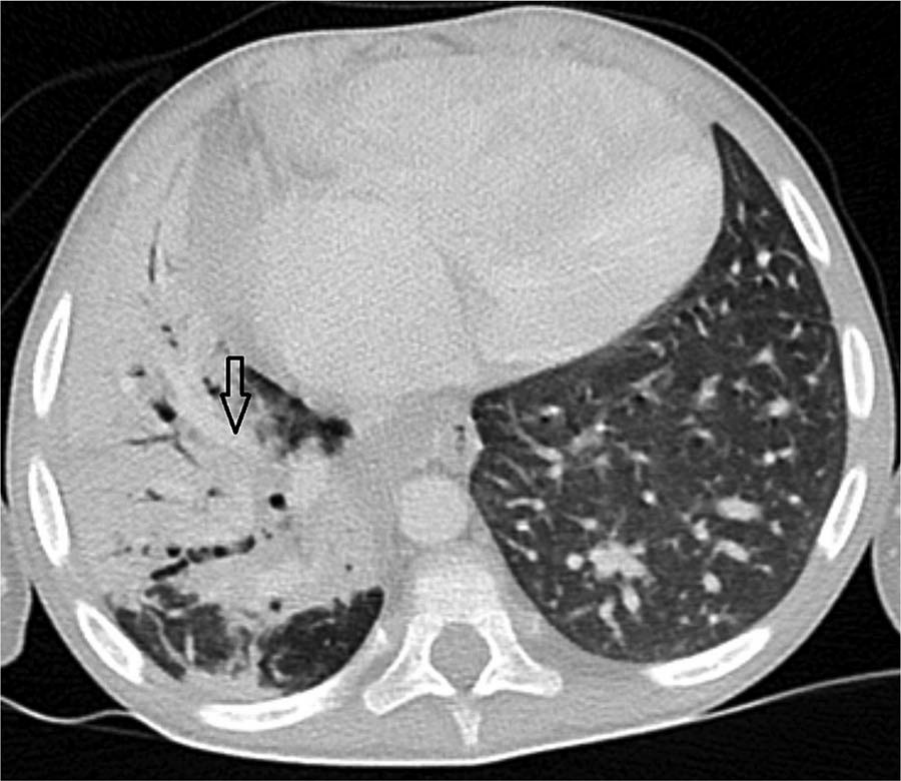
Axial CT angiogram in lung window demonstrating right lower-lobe consolidation and volume loss with mediastinal shift, consistent with pneumonia and compression by the pseudoaneurysm.
